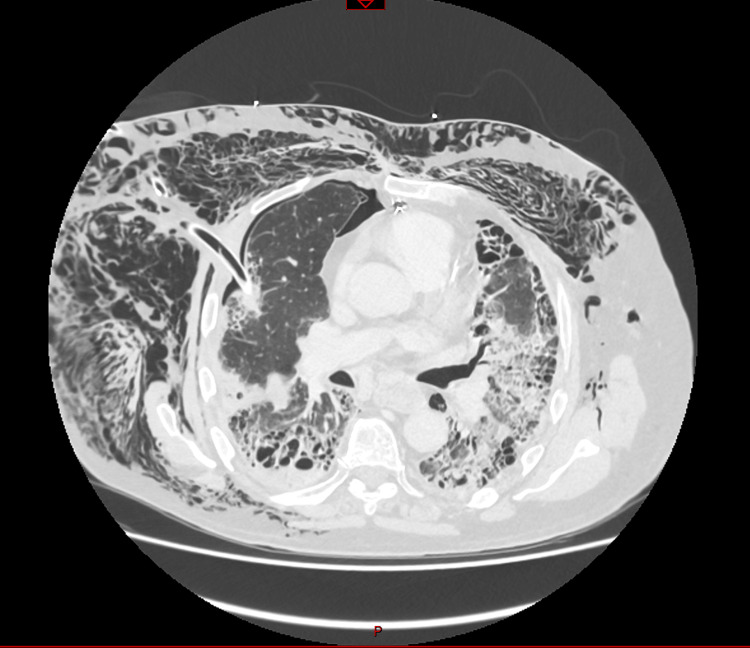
Chest drain malposition with penetration of the right lung: significant subcutaneous emphysema. Chest CT scan with contrast.
